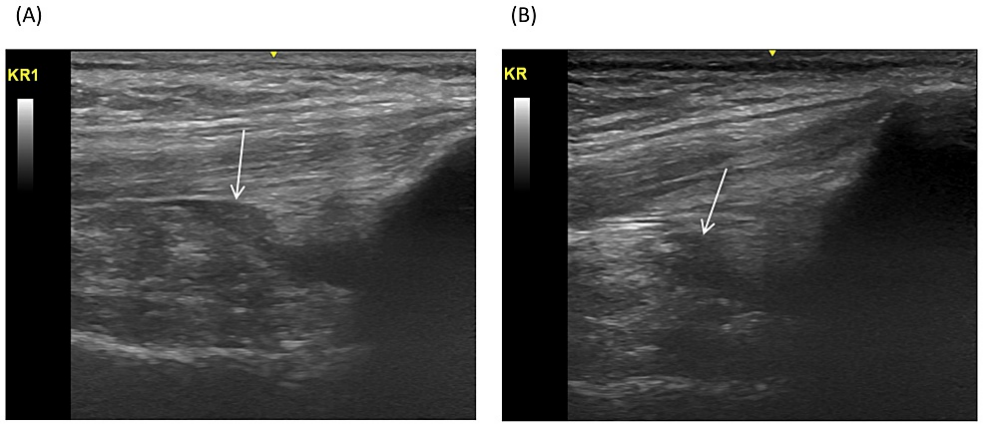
Ultrasonographic image of the right knee joint of Case 3 (A) showing bleeding (arrow) in September 2019 while receiving albutrepenonacog alfa (rFIX-FP) with 4000 units (59.7 IU/kg) once every two weeks, and (B) disappearance of bleeding (arrow) in May 2020 while receiving rFIX-FP with 4000 units (59.7 IU/kg) once weekly.
